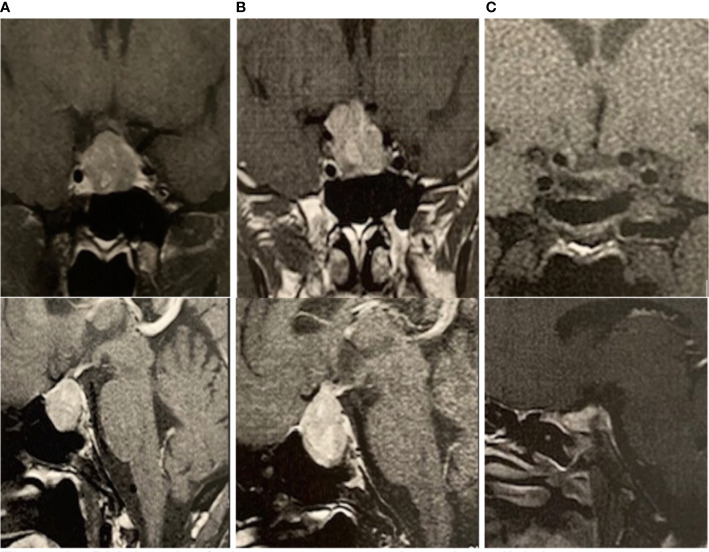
Coronal and sagittal MRI of individual II.1 (family 1). T1-weighted image with a 24 × 19 × 18 mm pituitary macroadenoma (A) that evolved after 6 months with hemorrhagic degeneration and compression of the optic chiasm (B). After surgery, a small remnant can be seen in the left half of the anterior pituitary gland (C).
